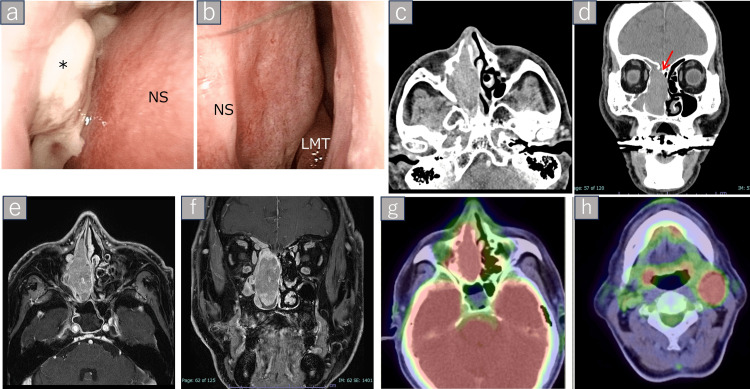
Preoperative endoscopic and imaging findings. The tumor is indicated by an asterisk (*) symbol. Endoscopic views of the nasal cavity. (a) Right nasal cavity showing a friable, hemorrhagic tumor occupying the nasal passage. (b) Left nasal cavity without evidence of tumor involvement. (b) Axial and (c) coronal CT images demonstrating bony erosion of the cribriform plate (arrow) and ethmoid sinuses. (d) Axial and (e) coronal contrast-enhanced MRI showing a heterogeneously enhancing mass extending from the nasal cavity to the anterior cranial base. (f) Axial and (g) coronal FDG-PET/CT demonstrating intense metabolic uptake in the primary tumor and left upper deep cervical lymph node, consistent with nodal metastasis. NS: nasal septum; LMT: left middle turbinate; FDG-PET: fluorodeoxyglucose-positron emission tomography
